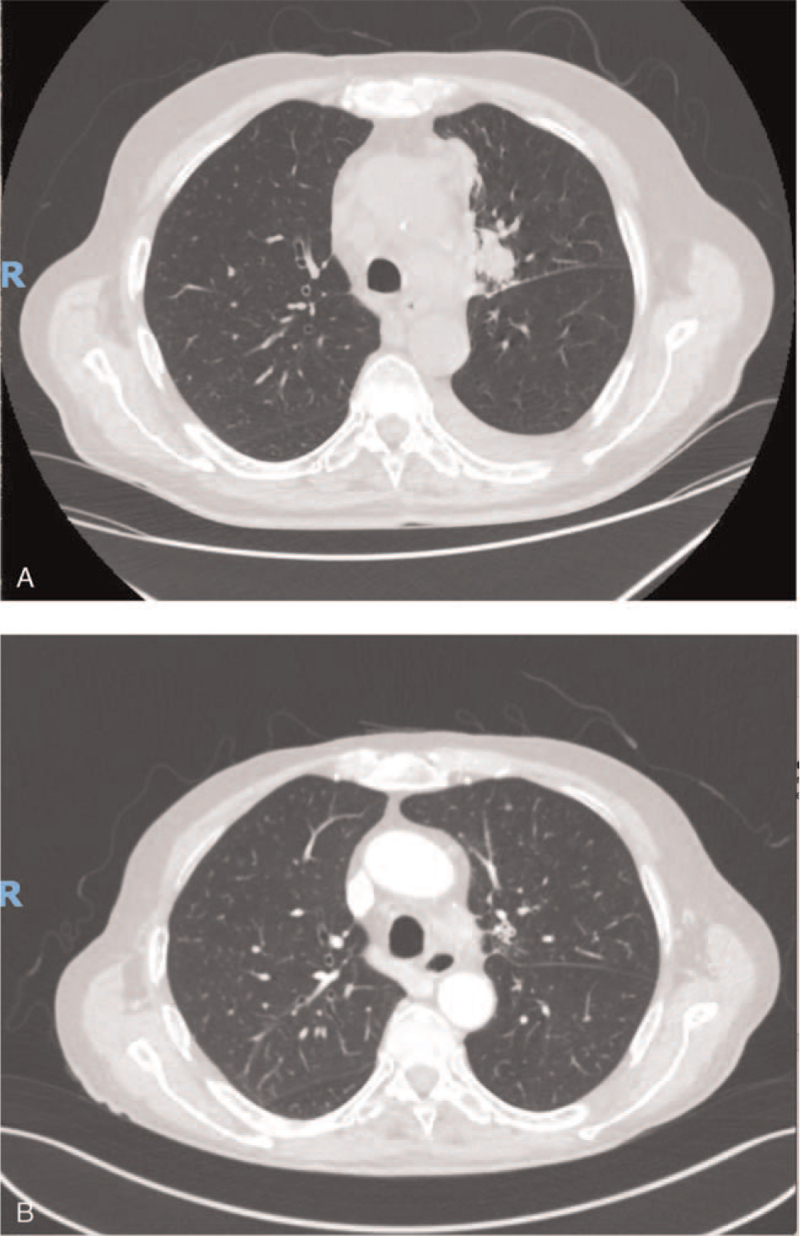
Tumor assessment by CT-scan at baseline (A) and after 6 months of treatment with alectinib (B). CT = computerized tomography.
